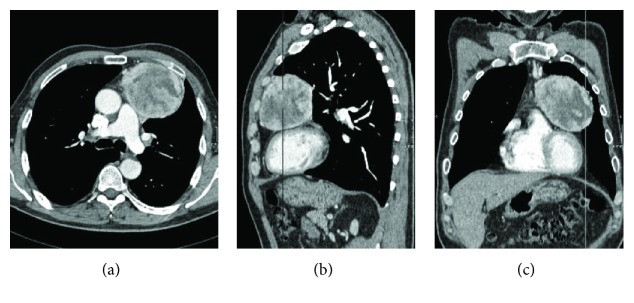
Contrast-enhanced CT of the chest. (a) Axial, (b) sagittal, and (c) coronal reformatted images revealing a well-defined anterior mediastinal mass, abutting the heart showing heterogeneous enhancement with pericardial invasion without any evidence of myocardial, aortic, or pulmonary artery involvement.
